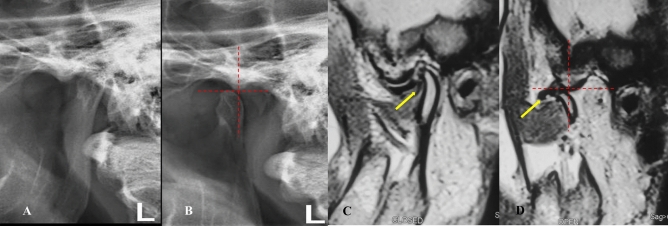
Lateral TMJ view represents stage I-C in closed (A) mouth position and in open (B) mouth position, showing the condyle located anterior to the eminence but at the same level as the highest point of the eminence. Oblique sagittal T1-weighted images represent Stage I-C, showing anterior disk displacement (yellow arrow) in closed (C) mouth position without recapture of the articular disk (yellow arrow) during open (D) mouth position. The disk-condyle complex remains at the same level as the highest point of the eminence
